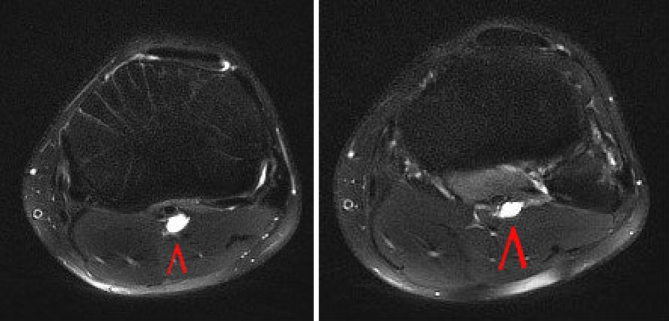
Axial MRI images of the left knee display peripheral enhancement of the tubular cystic mass involving the tibial nerve within its sheath extending from the popliteal fossa to the gastrocnemius muscle bellies, (red arrow).
